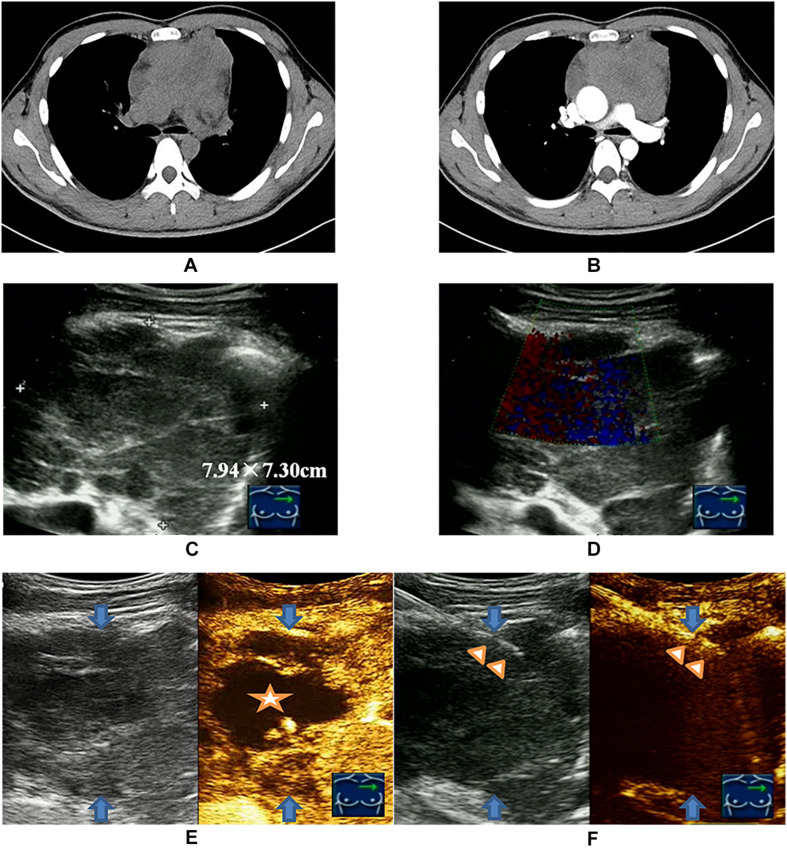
Viable-targeting ultrasound guided core needle biopsy for a 28-year old man with anterior mediastinal mass suspicious of B-NHL with insufficiency of sample for ancillary studies. (A) Plain CT scan showed irregular anterior mediastinal mass. (B) Contrast enhanced CT showed non-enhanced necrotic area interiorly. (C) Ultrasonography showed the anterior mediastinal mass with a size of 7.94 × 7.30 cm by the approach for the parasternal third inter-costal space. (D) CDFI was not applicable due to the heartbeat. (E) CEUS showed the non-enhanced necrotic area (indicated by the star symbol) and enhanced viable area definitely (peripheral of upper right) of anterior mediastinal mass (blue arrows indicated). (F) US-CNB (triangle showed the needle) targeting the enhanced viable area revealed primary thymic large B cell lymphoma of anterior mediastinal mass (blue arrows indicated).
